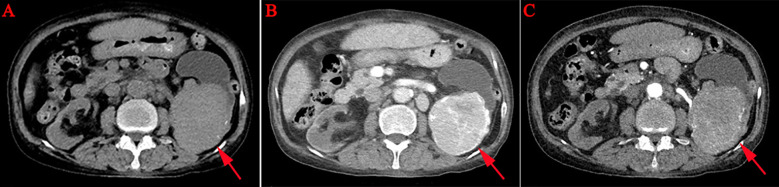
Computed tomography. These images revealed a massive soft tissue density mass in the left kidney, measuring approximately 8.5 cm × 6.4 cm, with clear boundaries, an intact capsule, and its inner edge protruding into the renal sinus, compressing the adjacent renal calyces, showing heterogeneous enhancement [(A–C), red arrow].
